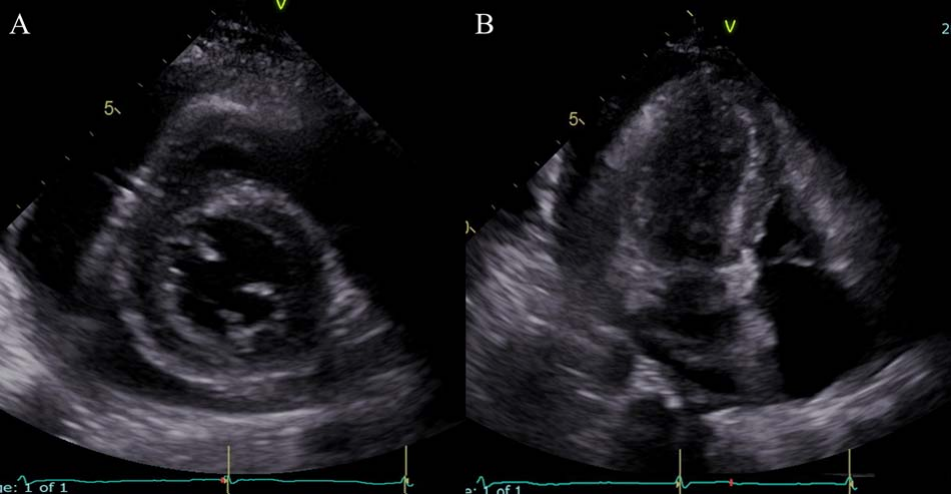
TTE at the time of admission; (A) Parasternal short-axis view: pericardial effusions of 7 mm around the right ventricle and 18 mm around the LV; (B) modified apical four-chamber view; TR insufficiency is unclear.
